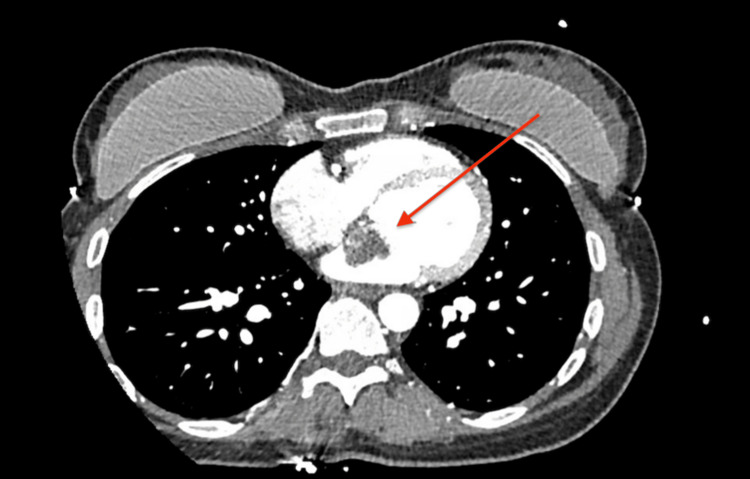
CT cardiac angiogram showing a 30 mm (red arrow) filling defect arising from the anterior wall of the left atrium, in keeping with the known history of myxoma.
